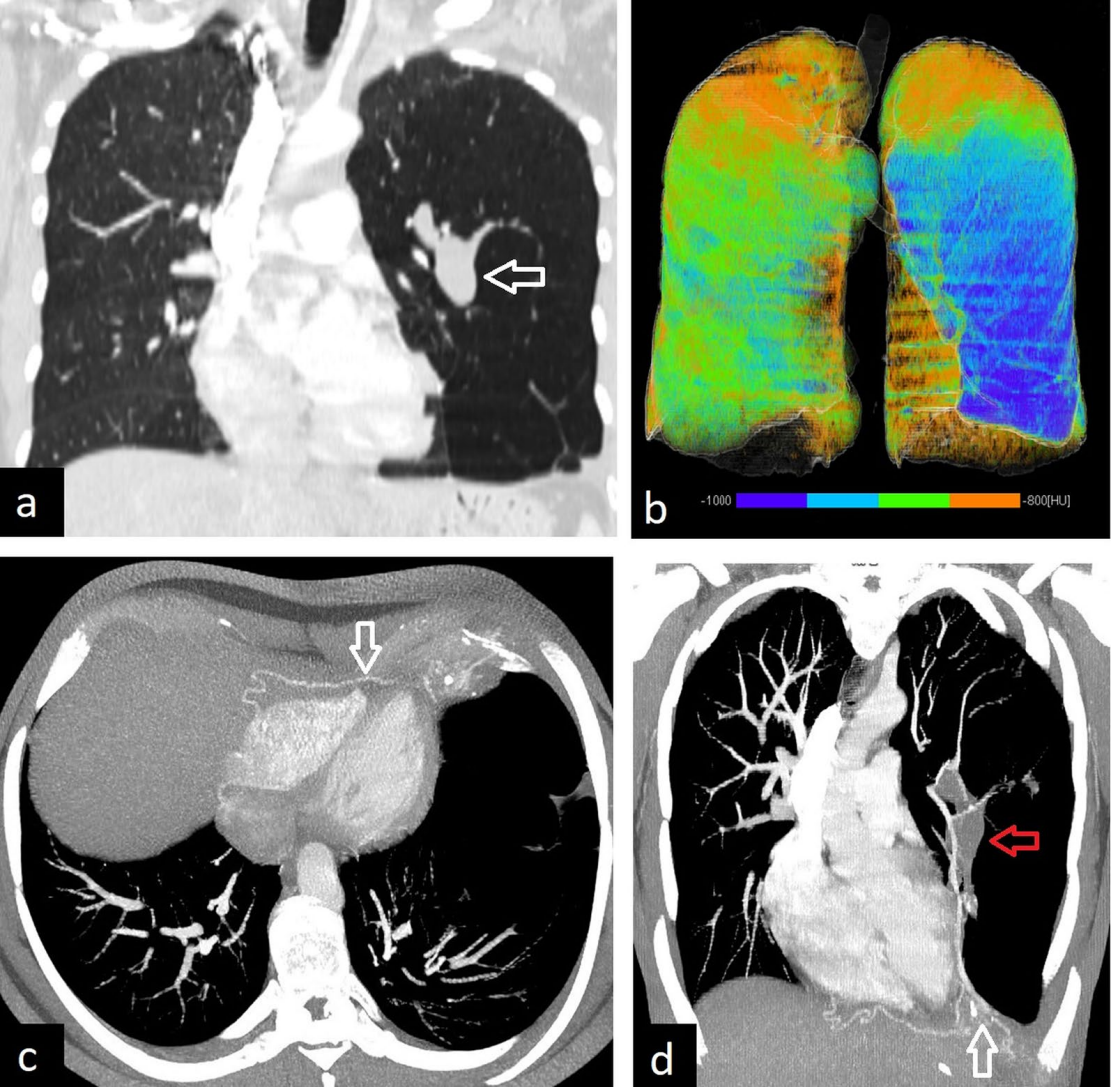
Mixed lesions-pulmonary sequestration and bronchial atresia in a 45-year-old asymptomatic female. Coronal CT image shows bronchial atresia with the finger-in-glove appearance (a, arrow). Hyperinflation of the obstructed lung area is observed on both coronal (a) and quantitative 3D reconstruction CT images (blue area) (b). Axial (c) and coronal (d) maximum intensity projection (MIP) images demonstrate pulmonary sequestration supplied from an aberrant artery arising from the right inferior phrenic artery (arrows). Bronchial atresia is also seen on the coronal MIP image (red arrow)
